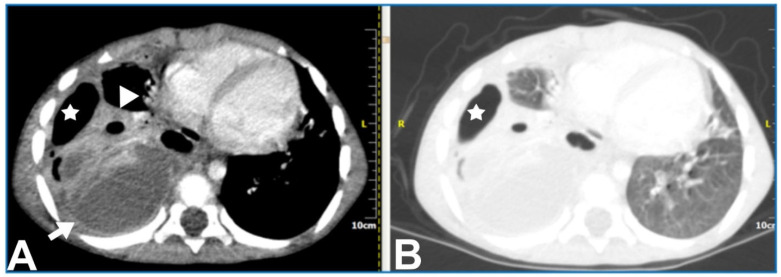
A 5.5-year-old boy with complicated pneumonia underwent a CT scan because of a differential diagnostic issue between empyema and lung abscesses on CR and LUS. Axial chest contrast-enhanced CT scan with soft-tissue window (A) and lung window (B) depicts a large multiloculated empyema (arrow), collapsed lung parenchyma with cavitary necrosis (arrowhead), and loculated pneumothorax (stars). Further, optimal treatment was drainage of the empyema based upon the chest CT findings.
